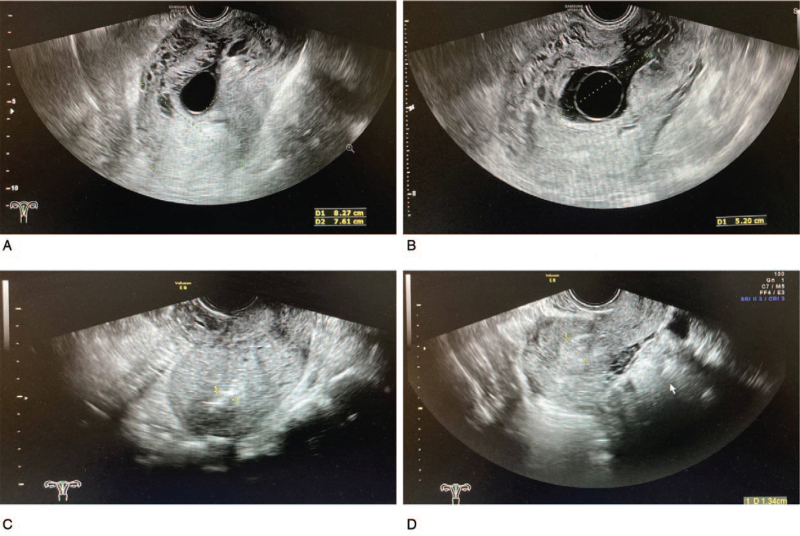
CSP ultrasonography. A. Transvaginal ultrasonography revealing CSP before D&C. B. Transvaginal ultrasonography showing CSP before H/S. C. Transvaginal ultrasonography showing CSP after D&C. D. Transvaginal ultrasonography showing CSP after H/S. CSP = caesarean scar pregnancy, D&C = dilation and curettage, H/S = hysteroscopy.
